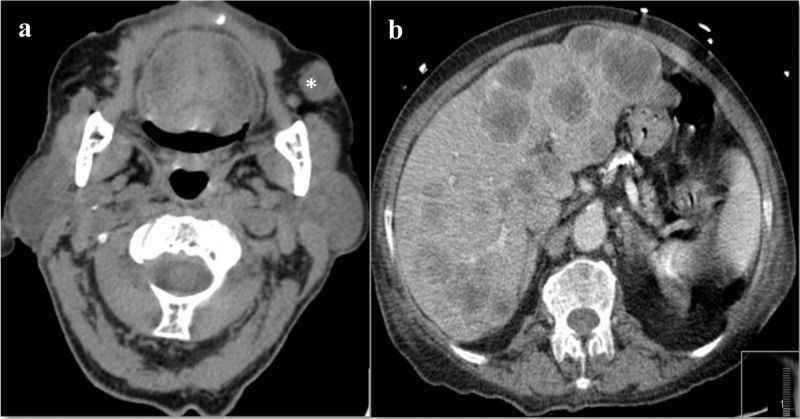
CT scan images at relapse of disease (5 months after diagnosis). Head CT scan (A) showing local tumor aggressiveness with infiltrative pattern (∗). Abdominal CT scan with multiple liver metastases.
